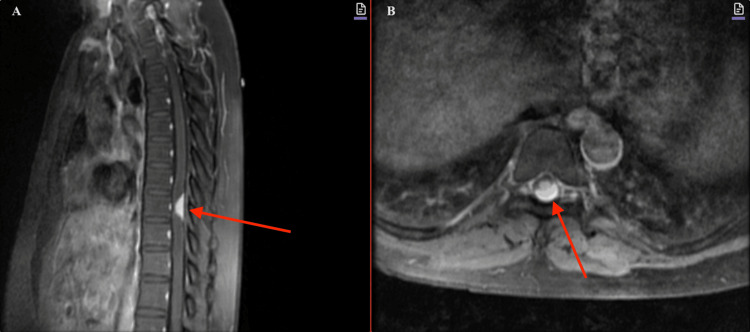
Preoperative T1-weighted contrast-enhanced MRI of the thoracic spine Sagittal (A) and axial (B) T1-weighted contrast-enhanced MRI images showing an intradural contrast-enhancing lesion centered at the T9 level (red arrows), causing severe spinal canal stenosis with spinal cord compression and edema.
